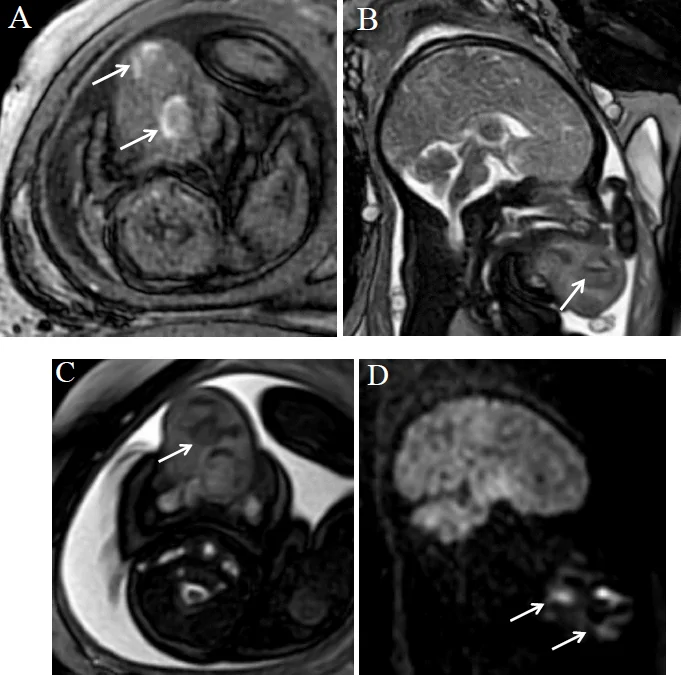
Prenatal MRI at 38 weeks. (A) Axial T1-weighted image shows a mass isointense to muscle with patchy hyperintense areas (arrow). (B, C) Sagittal and axial T2-weighted images show a mass slightly hyperintense to muscle with patchy hypointense areas (long arrow). (D) Sagittal diffusion-weighted image shows slightly heterogeneous hyperintense signal (arrow).
